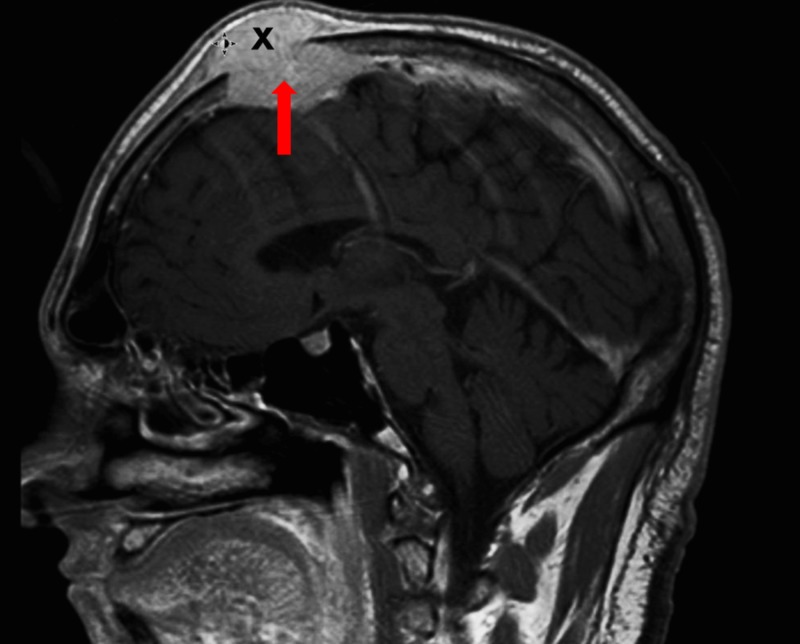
Magnetic resonance imaging of the head showing an enhancing calvarial mass (marked X) invading into the subcutaneous tissue (red arrow). Also visible is the involvement of the inner table of the calvarium, and invasion of the dura mater by the mass at the vertex.
